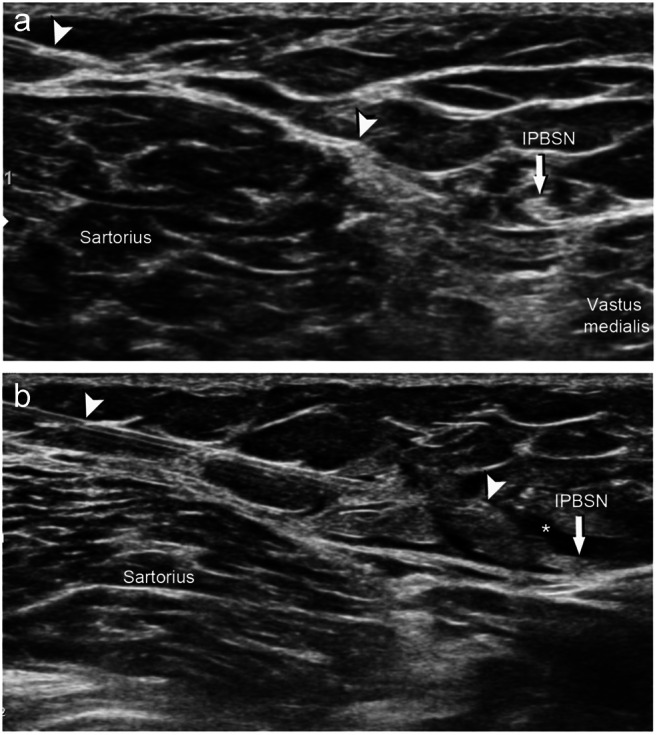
Ultrasound-guided perineural injection of the infrapatellar branch of the saphenous nerve (IPBSN; arrows) using a 25-gauge 60-mm cannula (arrowheads) before (a) and after (b) injection of 1 mL lidocaine 1% distributing around the IPBSN (asterisk)
